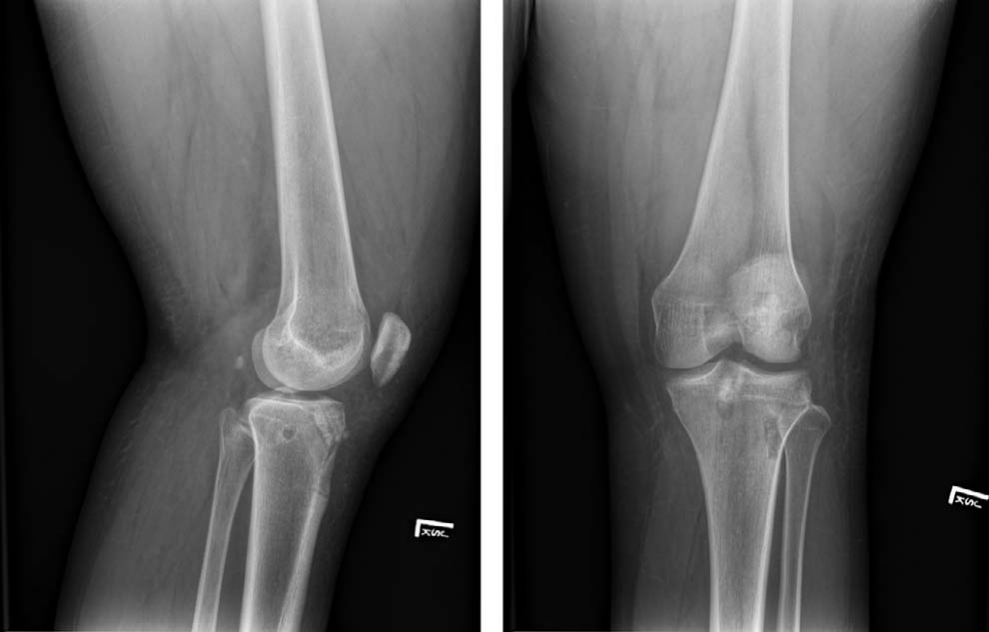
Lateral and standing plain radiographic views of the left knee show well-positioned femoral and tibial tunnels after anterior cruciate ligament reconstruction and normal patellar alignment.
